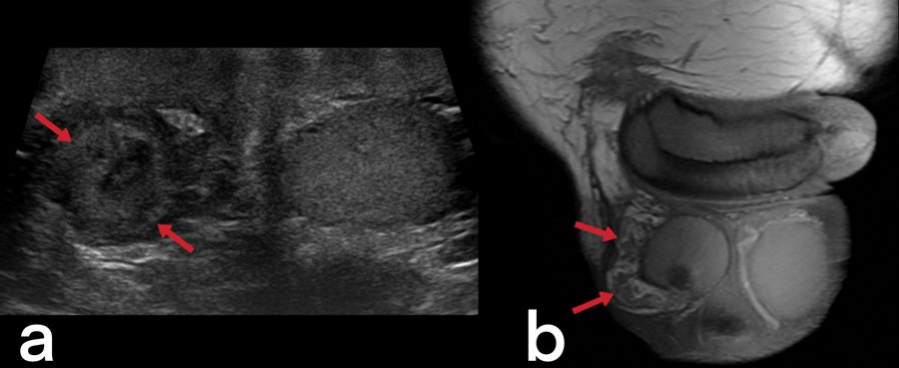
A 38-year-old male. Scrotal US image (a) and post-contrast coronal T1 weighted MR image (b) reveal diffuse heterogeneity, deformation, and dilated cystic lump with peripheral enhancement in the right epididymis (arrows)
